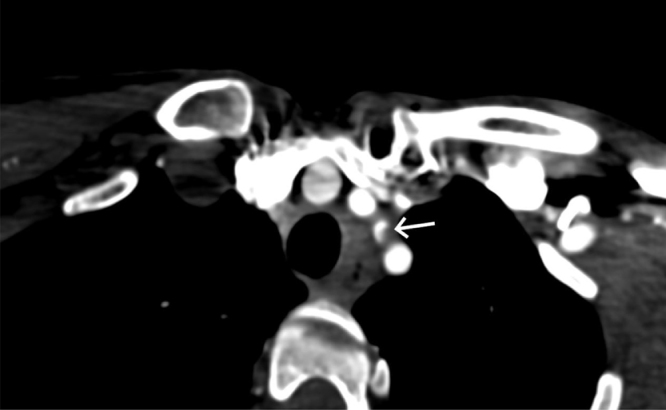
Axial computed tomography angiography image of the neck, demonstrating a partially occlusive intraluminal filling defect within the V1 segment of the left vertebral artery (arrow), consistent with vertebral artery dissection.
